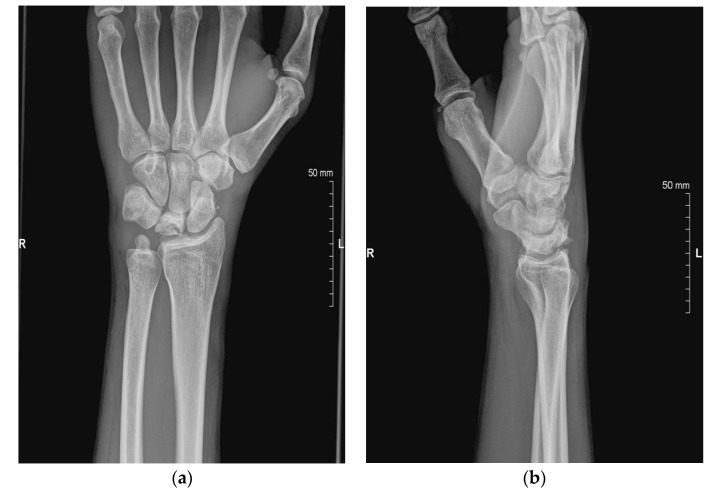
X-ray stage 3A: the collapse of lunate, carpal high preserved. (a) Stage 3A, AP view X-ray; (b) Stage 3A, lateral view X-ray.
